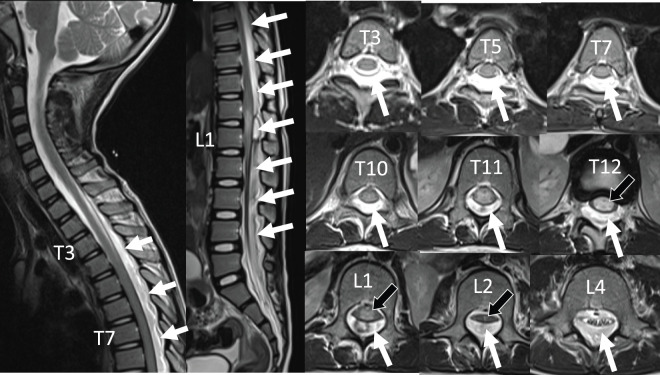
MRI, sagittal view and axil view in the T2 weighted images on 1 day after splenic detorsion. Spinal epidural hematoma was observed from T3 to L4 (white arrow) with spinal cord compression centered at T12-L1 (black arrow).
